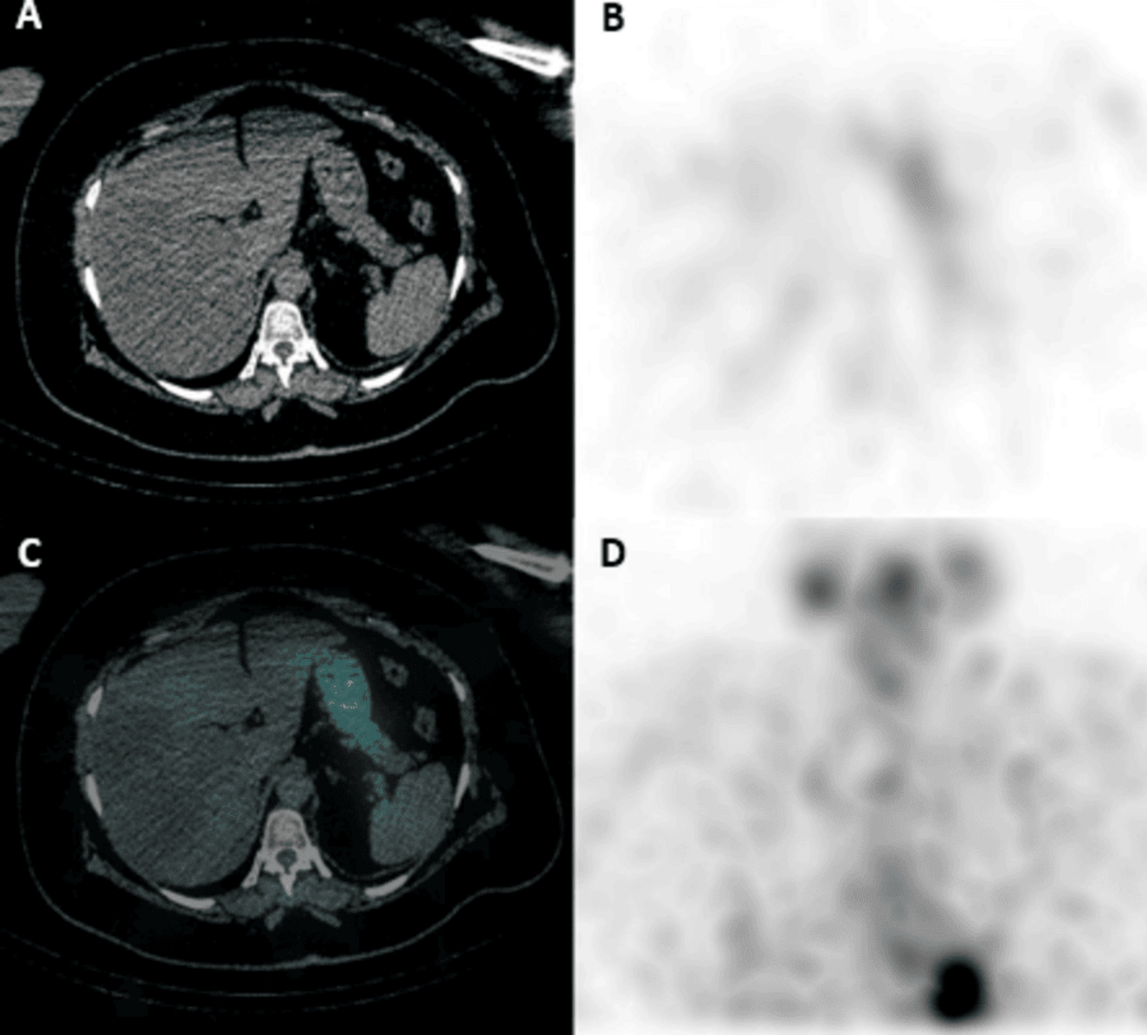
Diagnostic whole-body RAI SPECT/CT scan (second RAI dose). Diagnostic whole-body RAI SPECT/CT scan done six months after the second RAI dose does not currently show RAI uptake within the hepatic segment VII in SPECT, fused, and MIP images (B, C, and D, respectively). RAI, radioactive iodine; SPECT/CT, single photon emission computed tomography/computed tomography; MIP, maximum intensity projection
